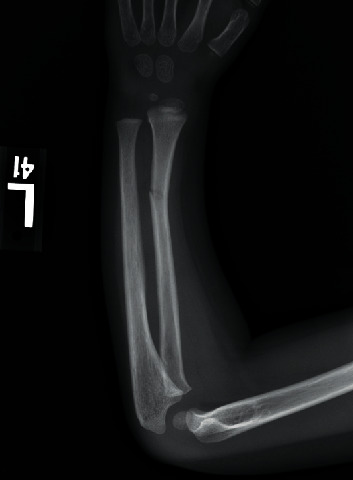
A radiograph demonstrating the left distal radius revealing a minimally angulated greenstick fracture.
